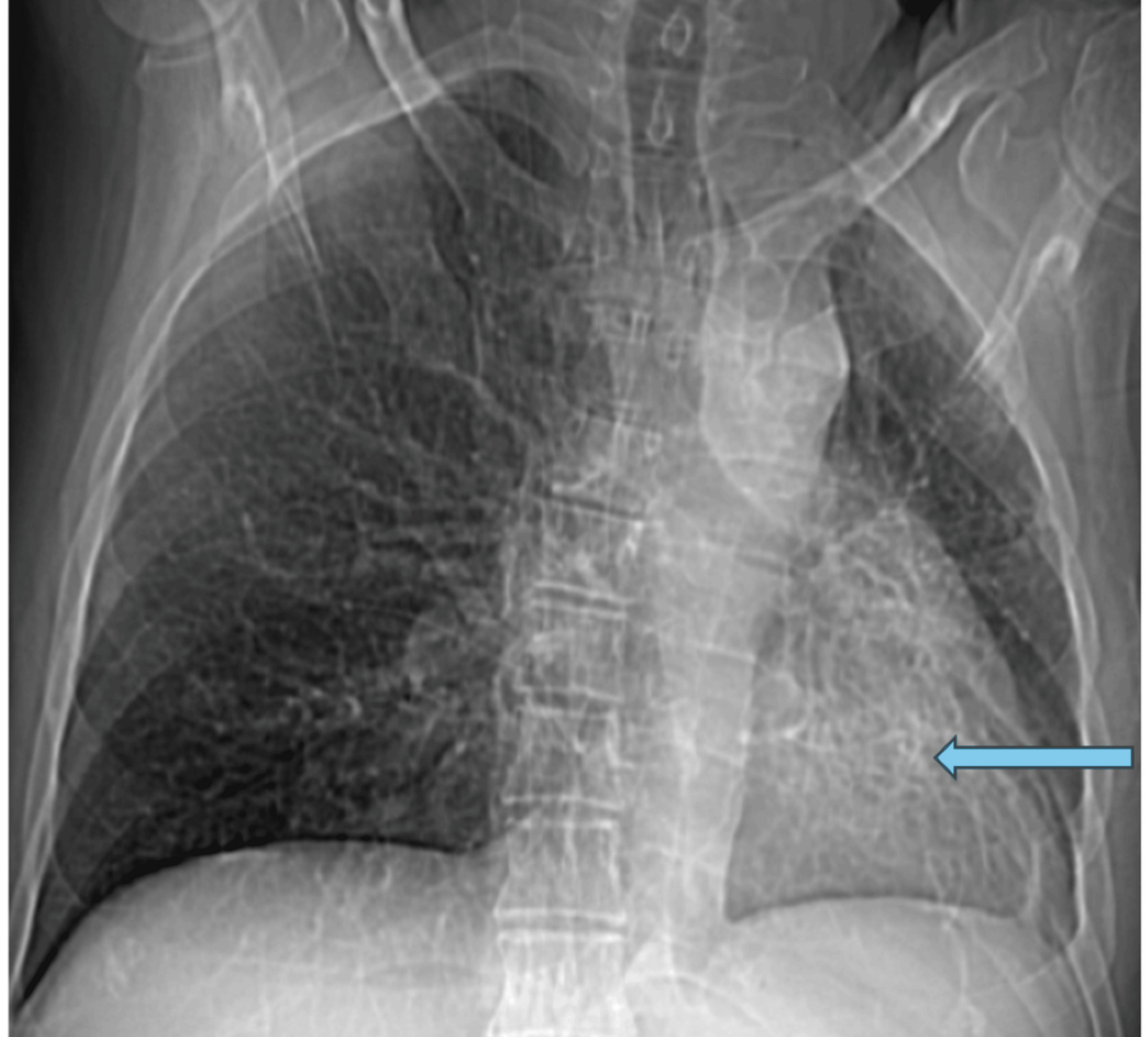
CT scout films show unilateral hyperlucency with small left lung and cystic bronchiectasis in the left lower zone lung fields.
